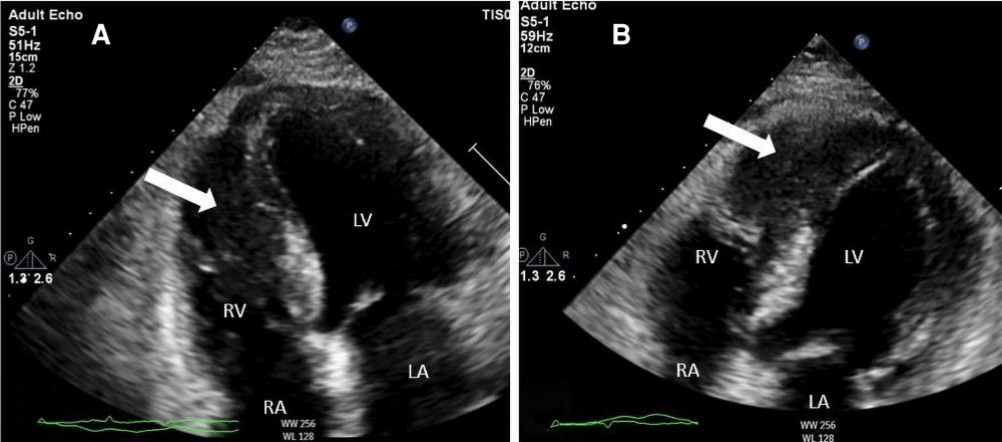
Transthoracic Echocardiography. An apical four-chamber (*A*) and right ventricular focused view (*B*) showing a large homogenous mass (white arrows) measuring 4.8 cm × 2.5 cm in the right ventricular apex and infiltrating the interventricular septum.
